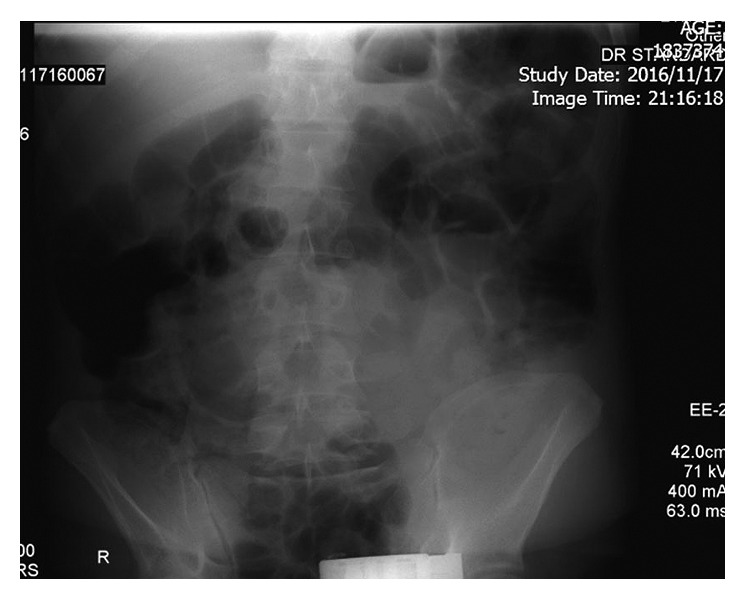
Plain abdominal X-ray during abdominal pain attack.
